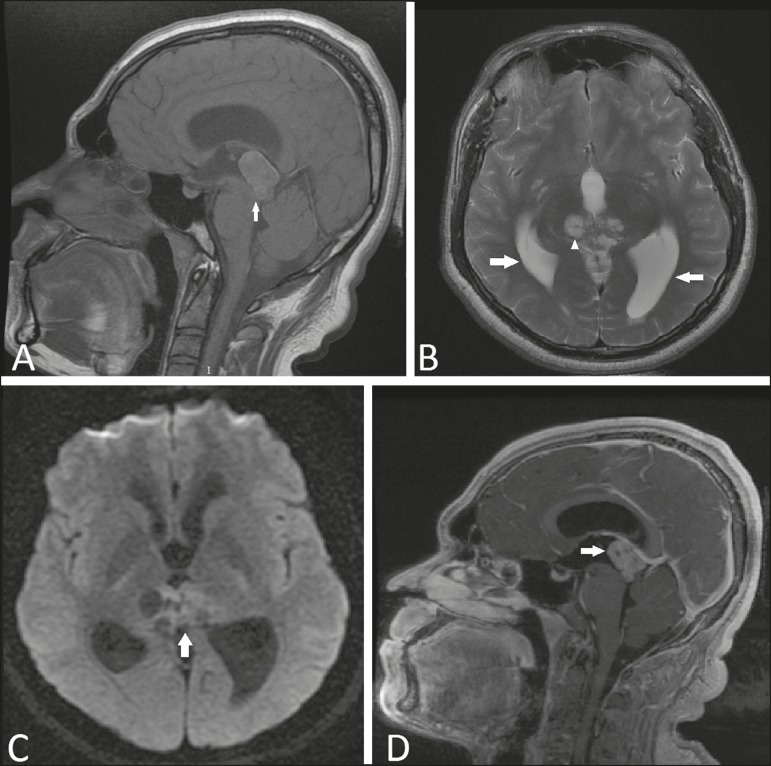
Magnetic resonance imaging. A: Non-contrast-enhanced sagittal
